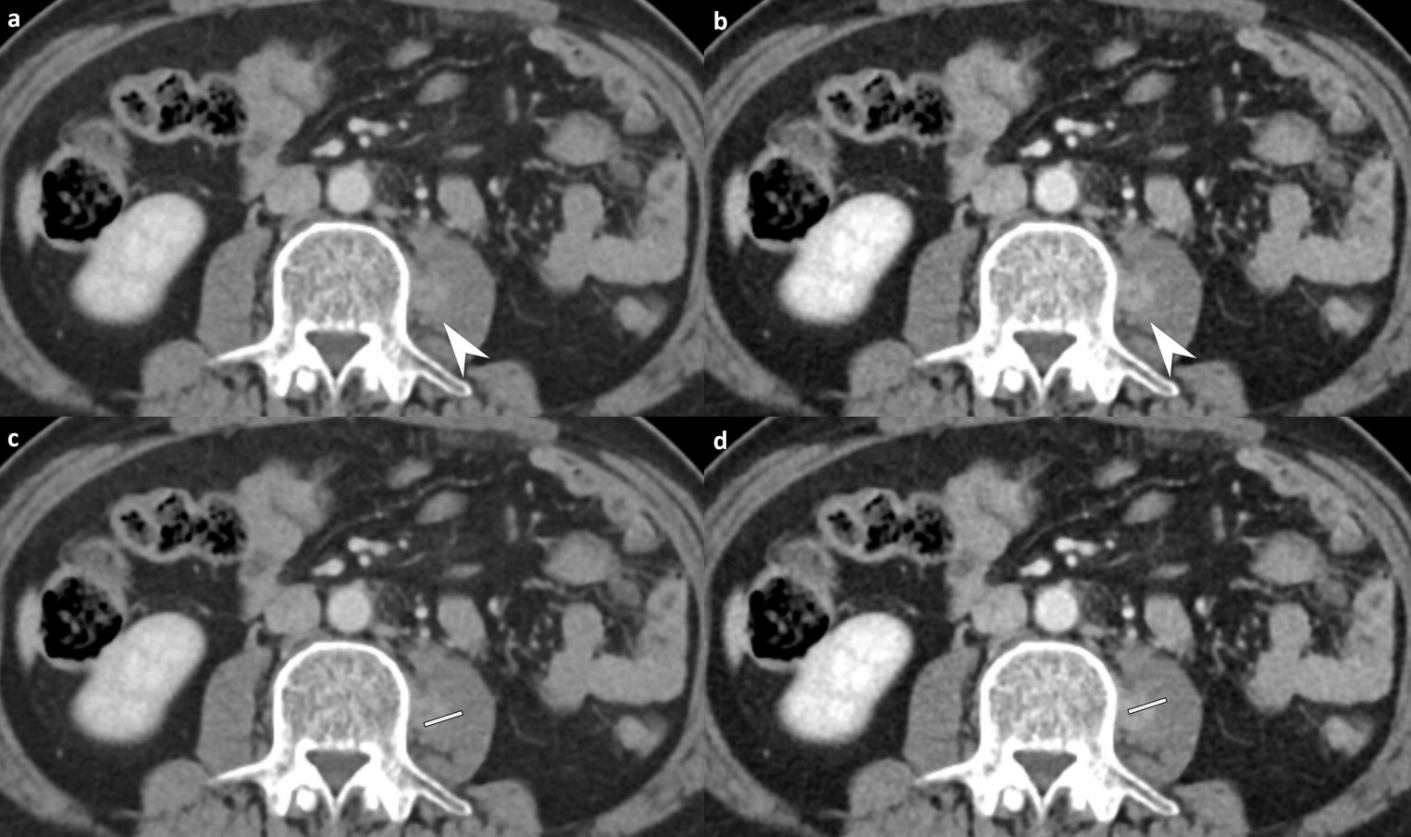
Contrast-enhanced abdomen CT in the portal venous phase in a 45-year-old man (body mass index, 24.8 kg/m^2^) with sigmoid colon cancer with a seeding nodule in the left psoas muscle. 80/Sn150 kVp CT (a-b, effective dose; 4.5 mSv) showing a hyperattenuating mass in the left psoas muscle (white arrows) by each reader (reader 1 and reader 2, grade 4 lesion conspicuity, grade 5 diagnostic confidence, and grade 5 overall image quality). The corresponding image obtained during the identical phase with 80 kVp CT (effective dose; 2.6 mSv) demonstrates markedly increased conspicuity of the lesion in the left psoas muscle by each reader (reader 1 and reader 2, grade 5 lesion conspicuity, grade 5 diagnostic confidence, and grade 5 overall image quality). Modified line-density profile revealed higher attenuation differences between the tumor and the normal tissue at 80 kVp (d) than those at 80/Sn150 CT (c) (maximum HU: minimum HU, 129:27 vs.106:36). CT, computed tomography.
